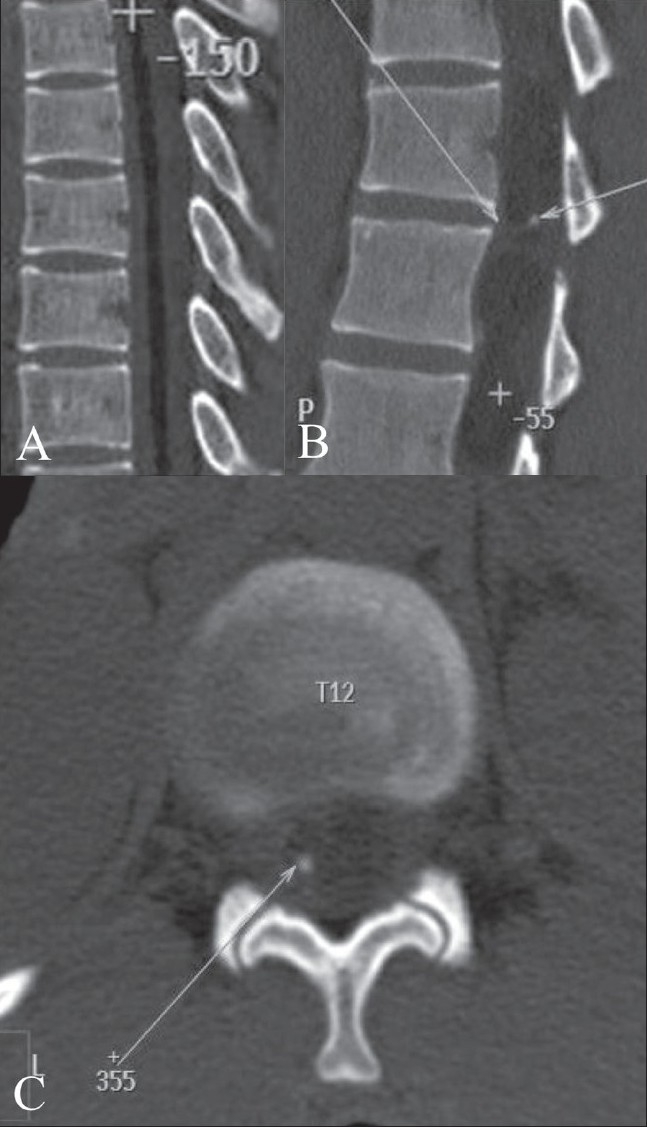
Non-contrast sagittal CT scan reconstructed images show intramedullary fat (+) in the upper spinal cord (A) and a mass in the conus region (long arrow) with areas of fat (+) and calcification (short arrow) (B). Noncontrast axial CT scan (C) shows a focal area of calcification (arrow) within the lesion.
